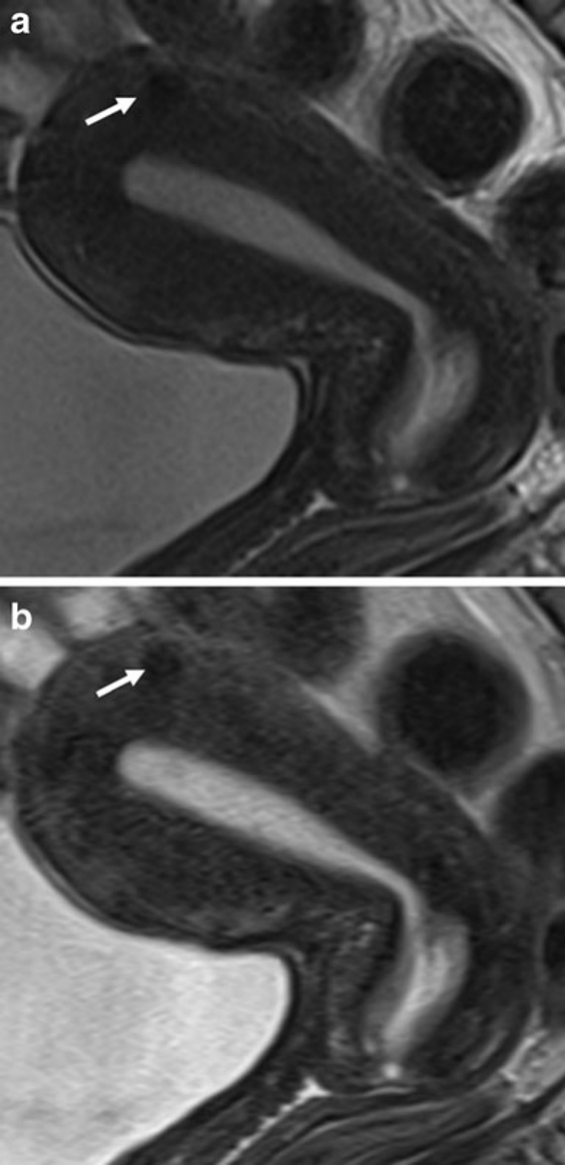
MRI of the normal anatomy of the uterus and cervix in a pre-menopausal 43-year-old patient referred to MRI for the assessment of an adnexal lesion (not shown). A small intramural leiomyoma is depicted (*arrows*). a Sagittal T2-weighted BLADE sequence. b Sagittal T2-weighted conventional TSE sequence. The uterine zonal anatomy (endometrium, junctional zone, myometrium) and the dorsal aspect of the bladder wall are more sharply delineated on the BLADE image (a); however, SNR and CNR are higher on the FSE image in b
